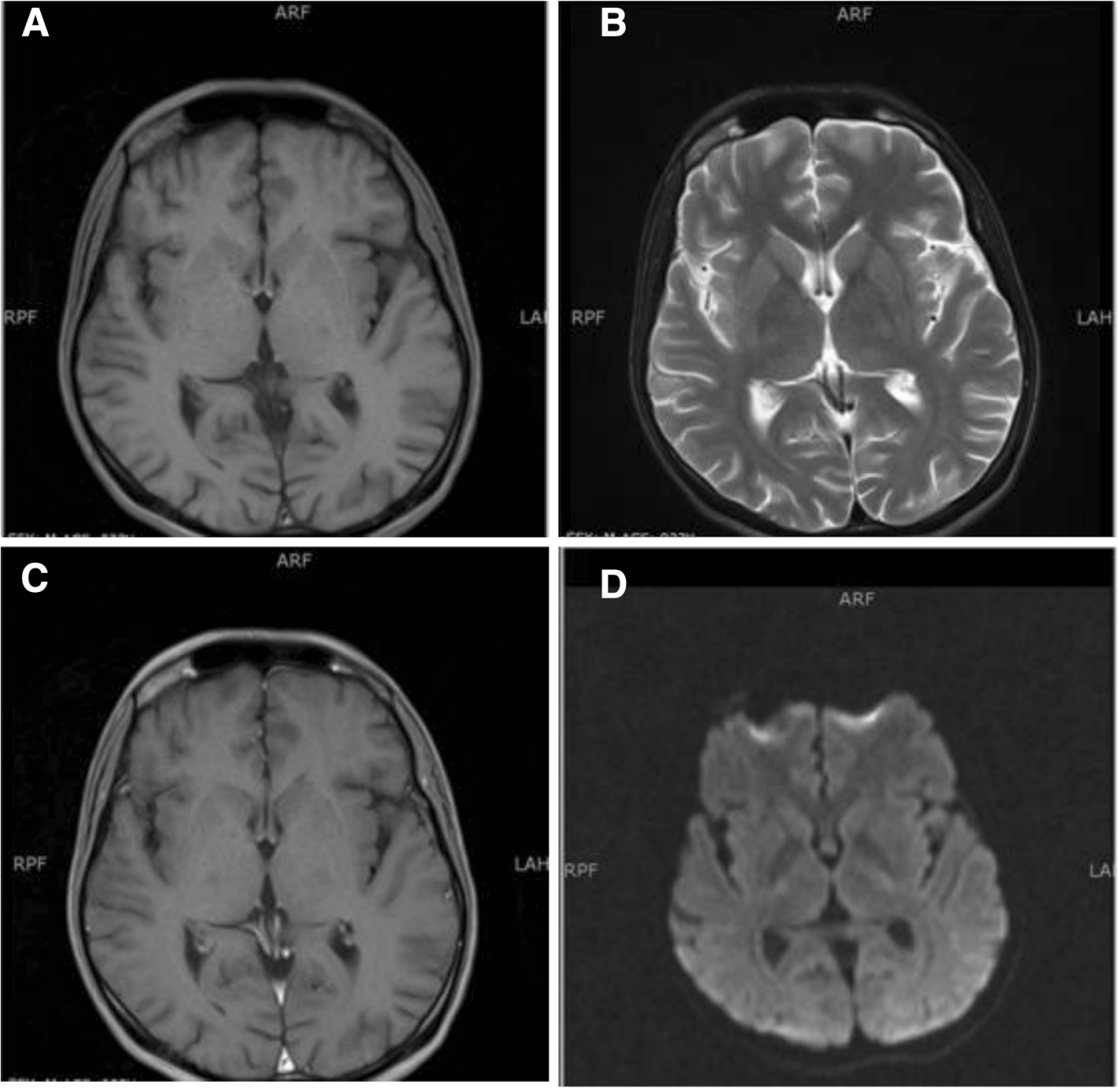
Magnetic resonance imaging scan of the brain six weeks after initial treatment with cisplatin. The changes of posterior reversible encephalopathy syndrome have resolved in both T1-weighted and T2-weighted images (A,B). The T1 post-contrast (C) and diffusion-weighted images (D) are also normal.
